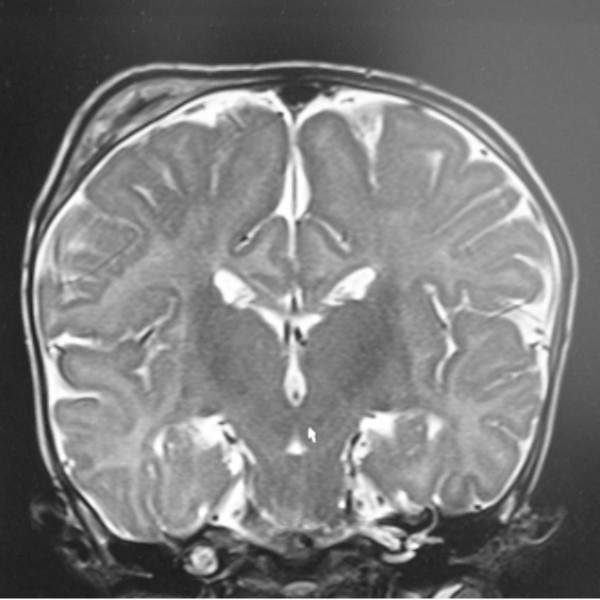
T_1 _weighted image of the skull showed expansile lesion within the parietal bone. The lesion is larger than appreciated on the radiograph. There is mixed hyper and hypointenstities. It is significantly expanded with well-defined margins.
